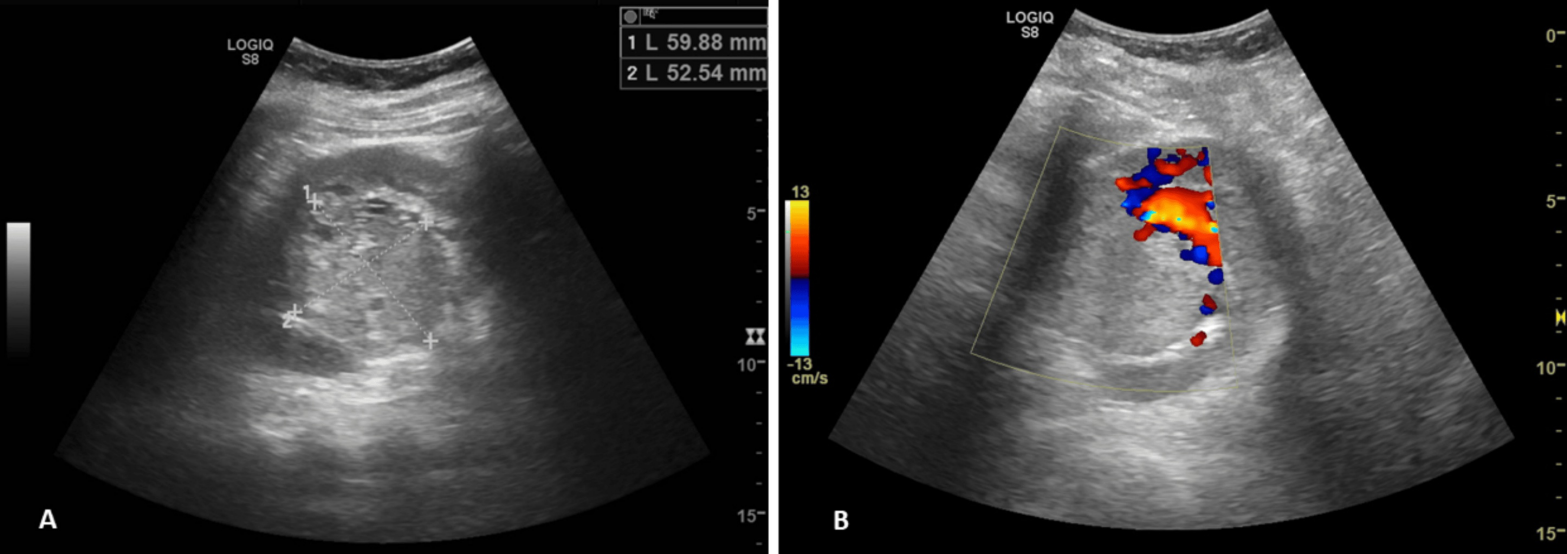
Ultrasound appearance of the renal mass (A) General appearance and dimensions of the renal mass; (B) Color Doppler revealing vascularization in the periphery of the lesion.
